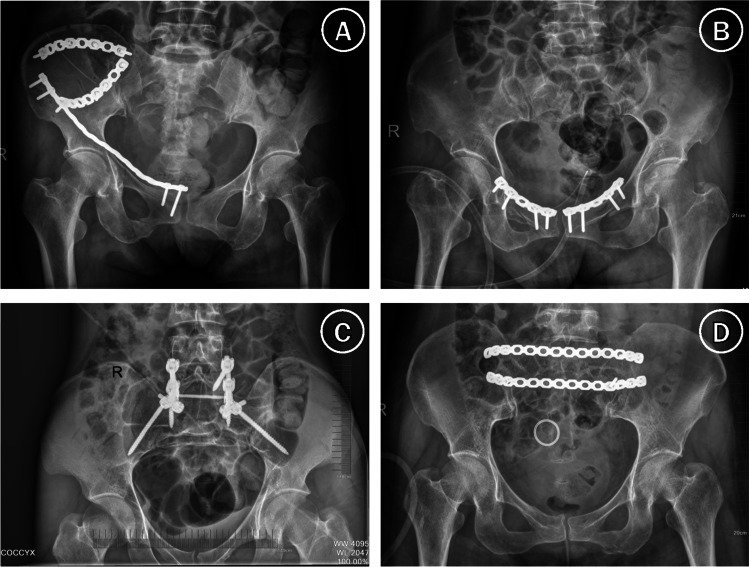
Postoperative pelvic X-ray images of nondisplaced pelvic fracture treated by ORIF. (A) Fixation of sacroiliac joint, ilium and superior pubic branch through anterior approach; first and third window of ilioinguinal approach. (B) Fixation of bilateral superior pubic branches via Stoppa approach. (C) Spino-pelvic fixation via posterior approach. (D) Ilioiliac plate fixation via posterior approach
